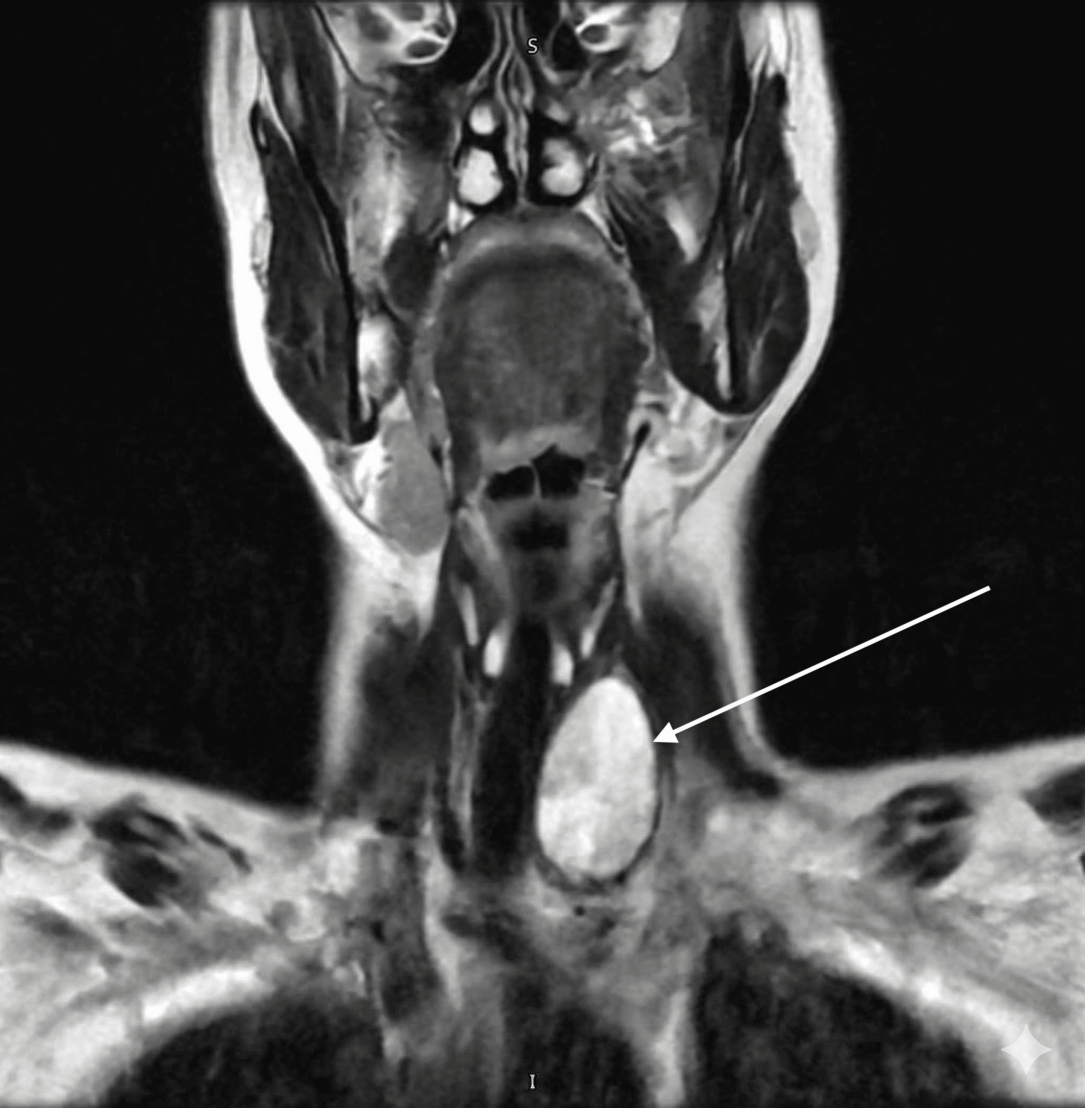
Cervical magnetic resonance imaging, T2-weighted coronal sequence. Cervical MRI, T2-weighted coronal sequence. The arrow indicates massive compensatory hypertrophy of the left lobe assuming total glandular function. Note the complete absence of the right thyroid lobe.
